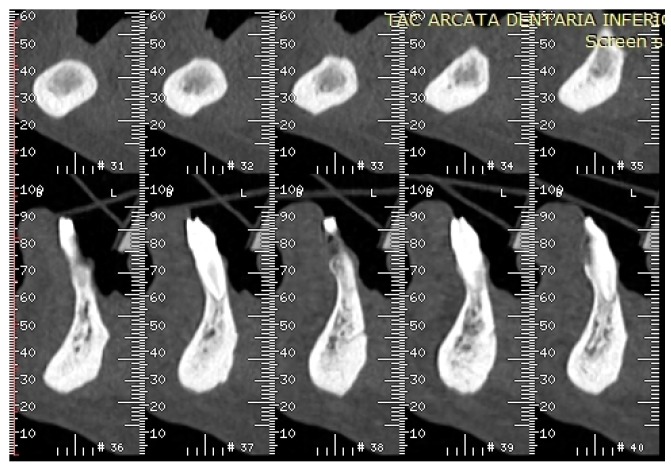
CT scan dated October 2016 showed no erosion of the mandibular buccal and lingual cortical bone.
